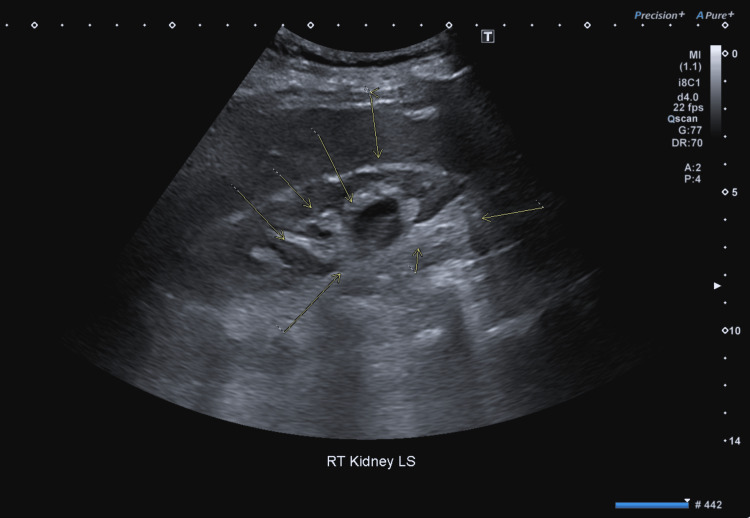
Renal ultrasound showing unilateral scarring Renal ultrasound demonstrated no acute abnormality. Incidental unilateral cortical scarring was noted.
